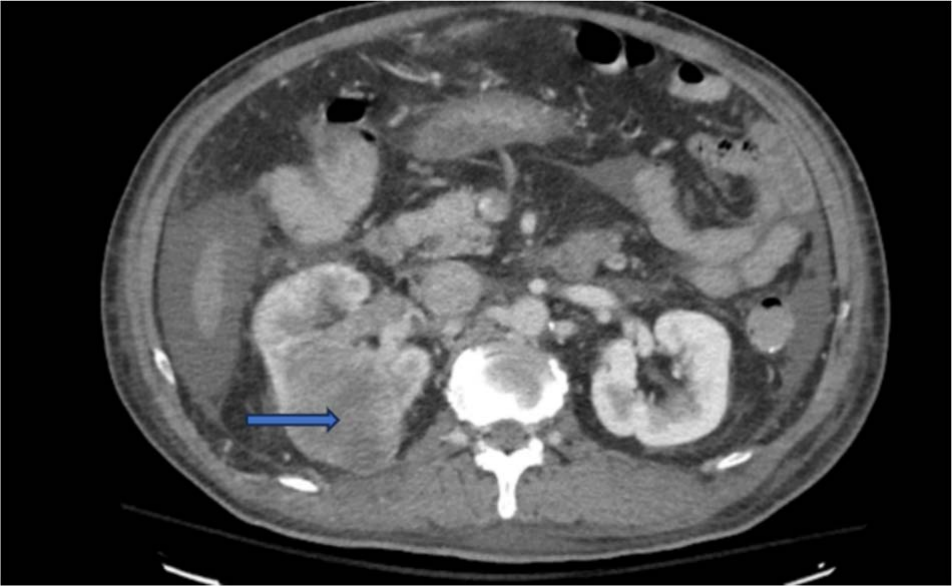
CT chest abdomen and pelvis with contrast showing middle portion of right with tumor during venous phase venous phase.
